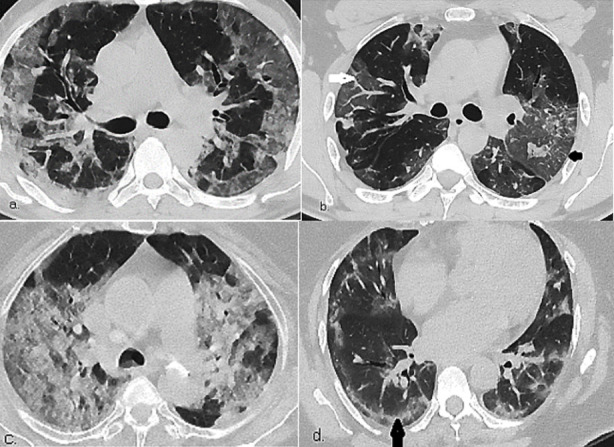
Axial HRCT Chest images of four different patients a) bilateral sub-pleural GGO and consolidation in posterior distribution b) Focal septal thickening within GGO (Black arrow). Vascular enlargement also seen (white arrow) c) Diffuse crazy-paving pattern and consolidation d) Sub-pleural line/band in peripheral distribution at late stage of disease.
